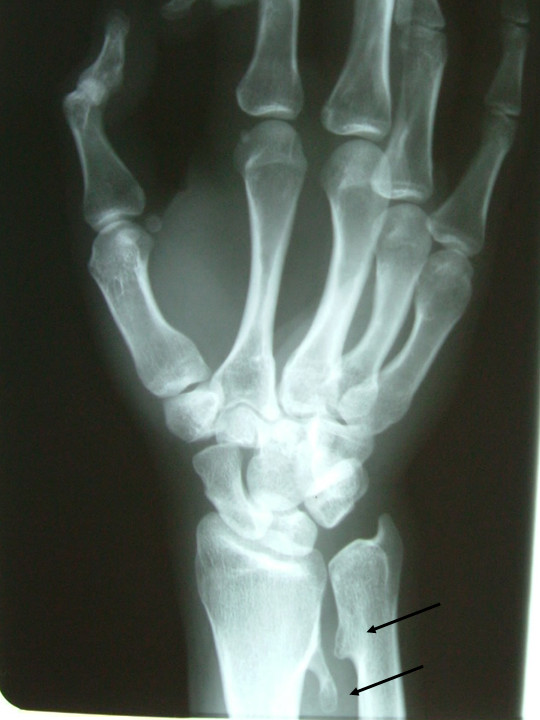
Oblique radiograph of the wrist showing the presence of two exostoses at the distal metaphysis of ulna and radius (arrows).
